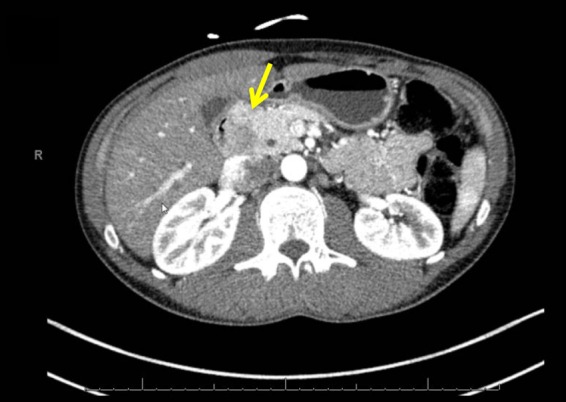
Computed tomography scan with the yellow arrow pointing at the tumor.
